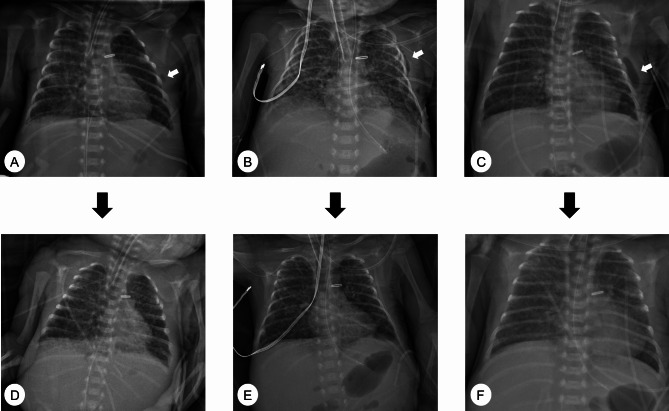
Chest radiographs showing subcutaneous emphysema (A-C, white arrow) around the thoracotomy site with full expansion of the left lung. The severity of subcutaneous emphysema was graded as scanty (A), mild (B), or moderate (C). All 19 patients’ subcutaneous emphysema was spontaneously absorbed (D-F) on the follow-up chest radiographs without active intervention
